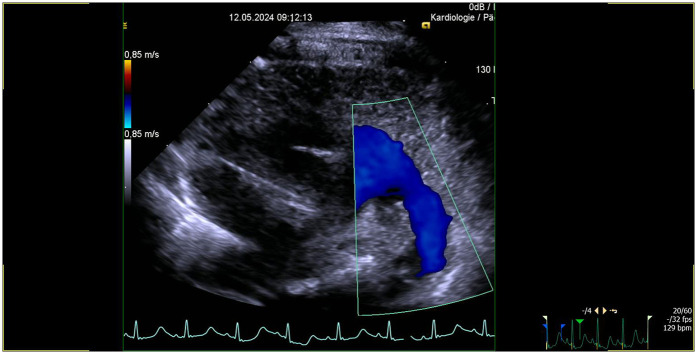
Transthoracic echocardiography, parasternal ductal view, of a predominantly right-to-left ductal shunt.
